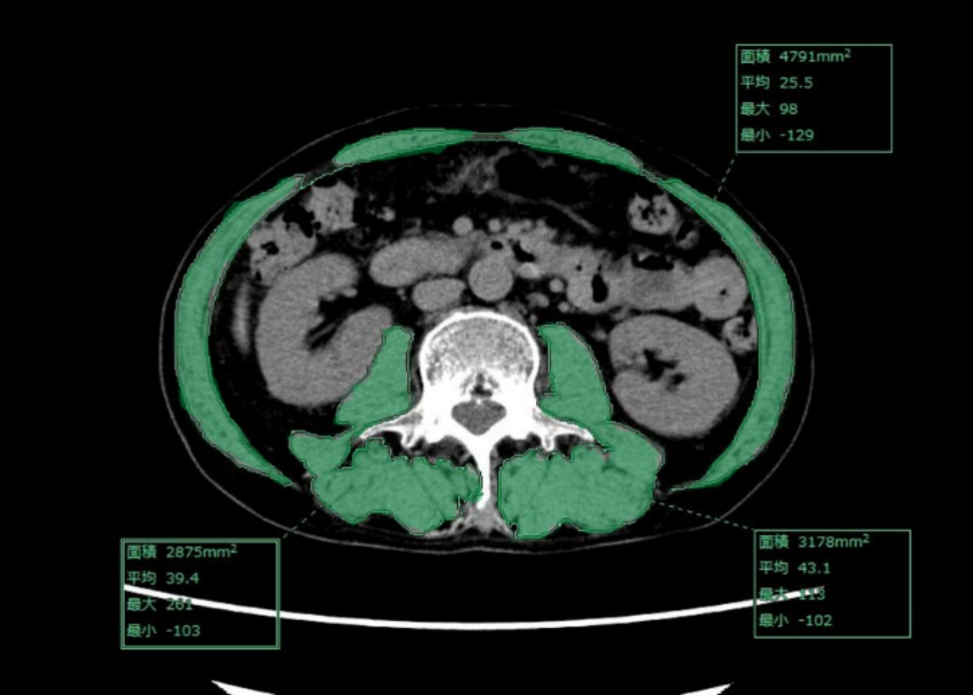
Axial computed tomography slice of the third lumbar vertebra (L3). Green areas indicate skeletal muscle
